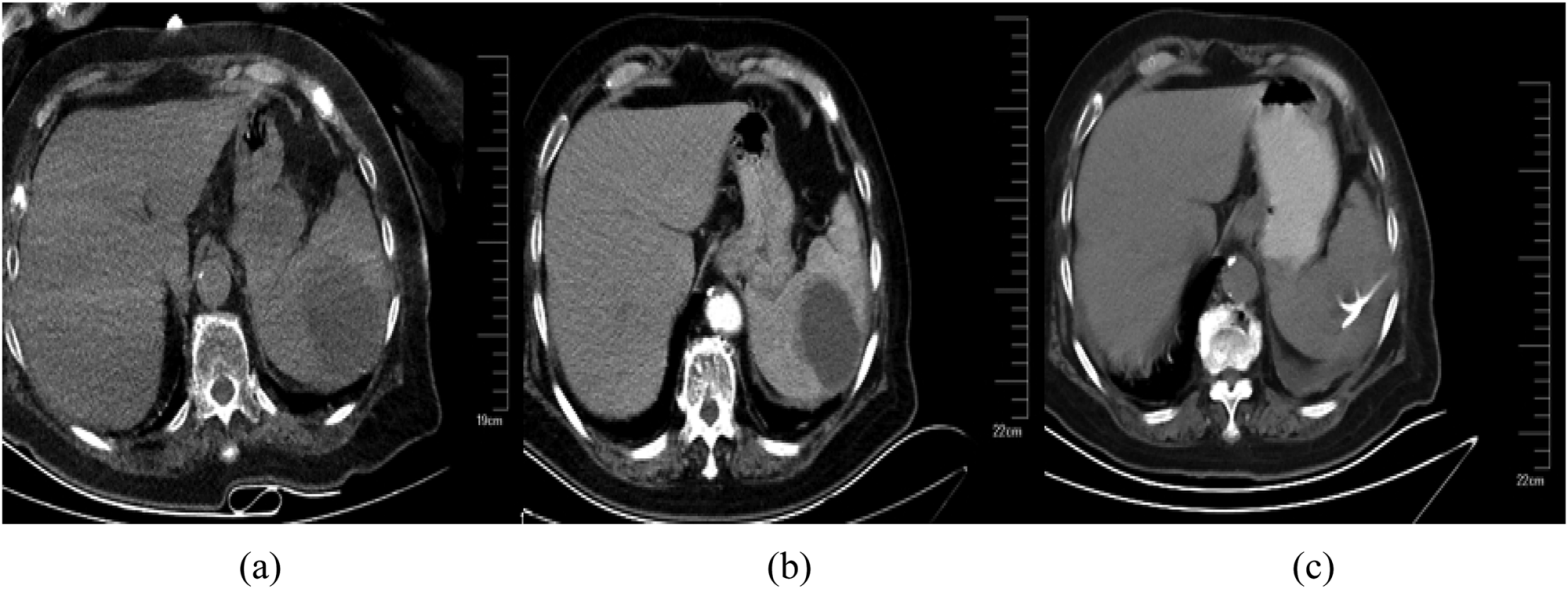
A CT scan of the abdomen revealed a low-density lesion in the spleen (a) before admission, (b) on day 5 after admission, (c) after paracentesis and drainage of the splenic abscess.
